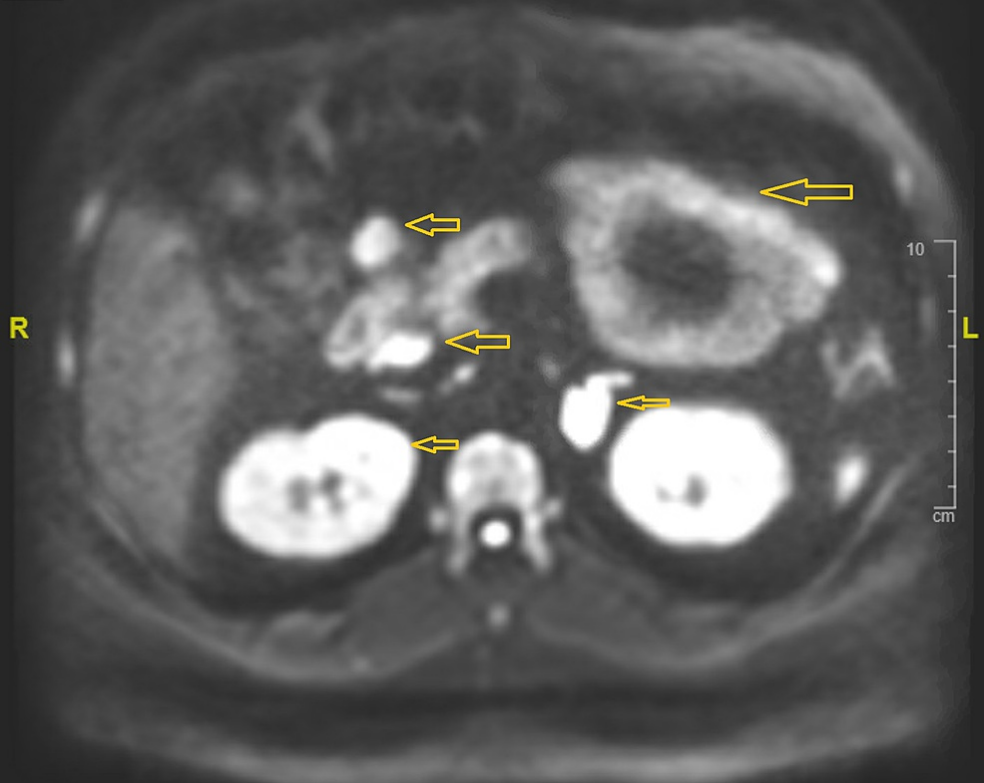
MRI abdomen with contrast showing multiple soft tissues enhancing nodules (yellow arrows) in the pancreas, duodenum, and bilateral adrenal gland
